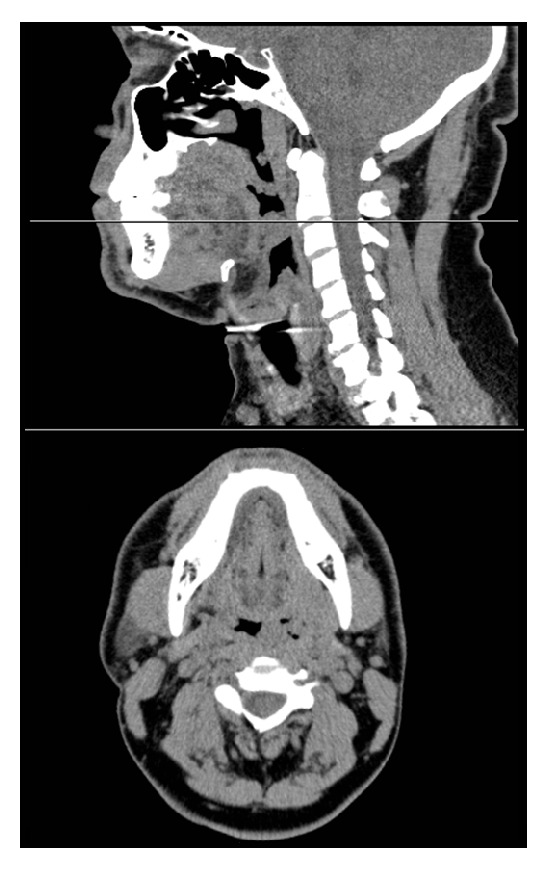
Noncontrast computed tomography. Sagittal and axial slices demonstrate a narrowed airway and thickening of the epiglottis (cursor shown for level) and subcentimeter cervical lymph nodes.
